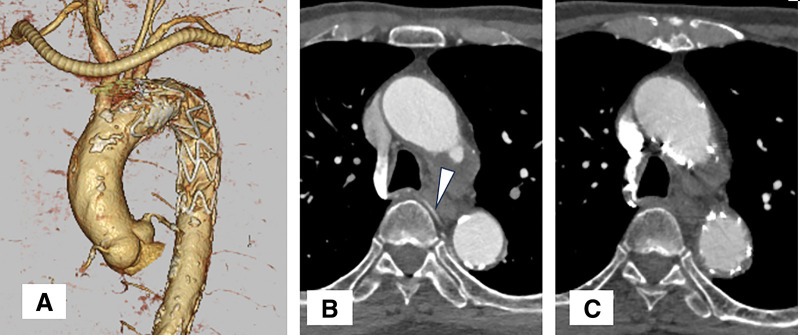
(A) The origin of the intercostal artery was broadly covered by the stent graft. (B) On the CT before TEVAR, the right 4th intercostal artery (arrowhead) was clearly identified. (C) On the CT after TEVAR, the right 4th intercostal artery could not be identified. TEVAR, thoracic endovascular aortic repair
